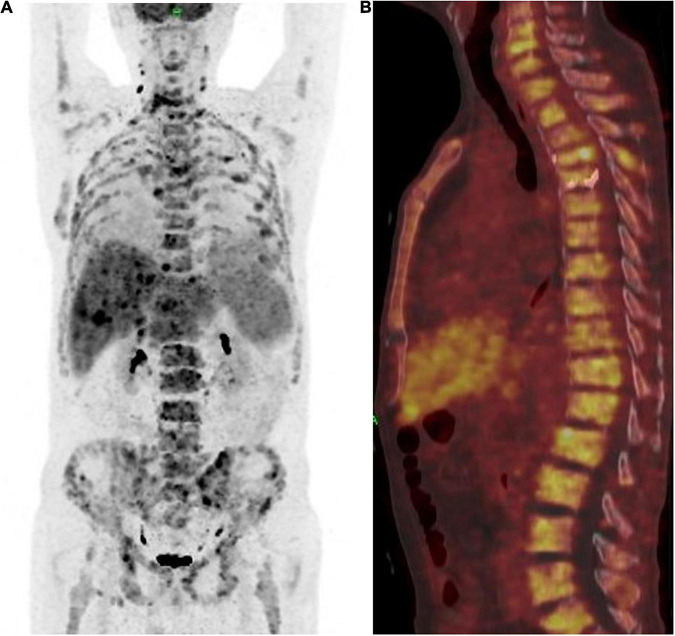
(A) Positron emission tomography and computed tomography showed the patient achieved partial response (PR) according to the mRECIST1.1 on metastatic lesions. (B) PET-CT showed a whole vertebra metastatic Primary EMPD, showing that a most obvious decrease of SUV from 18.9 to 5.3.
